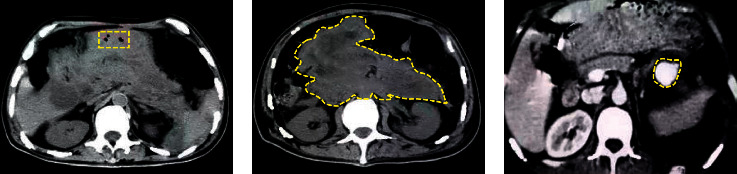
Three-dimensional CT images of patients with SAP. (a, b) The CT images of a patient with severe pancreatitis complicated with necrosis. (c) The formation of pseudocysts around the spleen in the patients.
